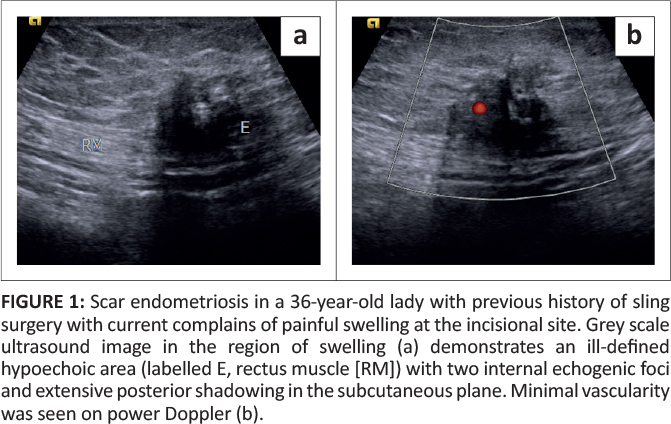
Scar endometriosis in a 36-year-old lady with previous history of sling surgery with current complains of painful swelling at the incisional site. Grey scale ultrasound image in the region of swelling (a) demonstrates an ill-defined hypoechoic area (labelled E, rectus muscle [RM]) with two internal echogenic foci and extensive posterior shadowing in the subcutaneous plane. Minimal vascularity was seen on power Doppler (b).
